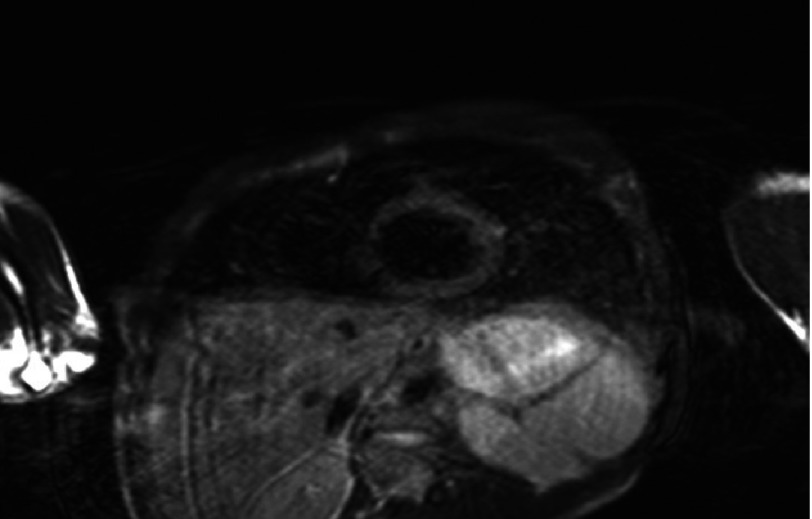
Cardiac MRI (Dark Blood T2WI) shows diffuse high signal intensity within the myocardium (arrow) representing a diffuse myocardial edema (acute myocarditis).
